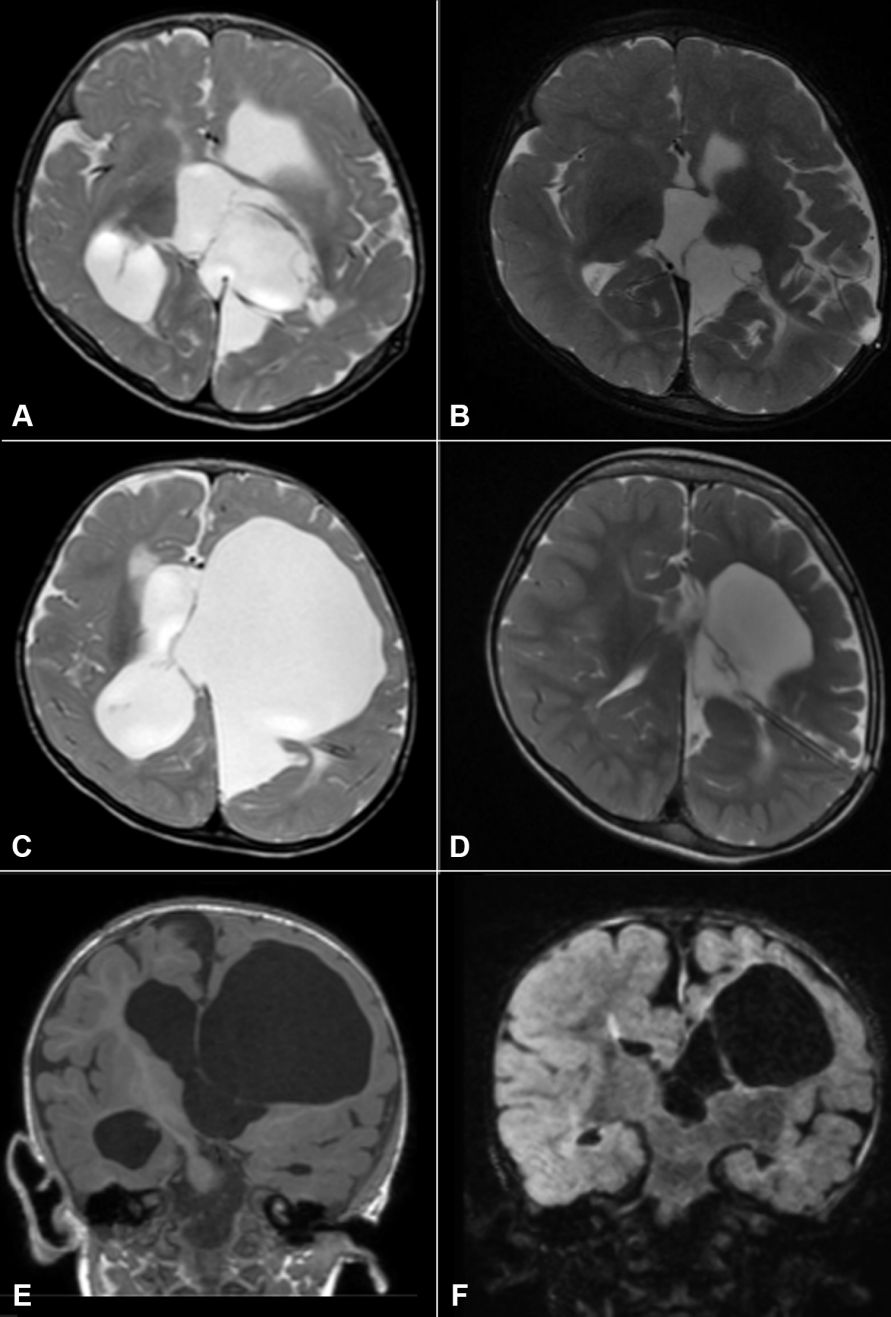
Axial T2-weighted (A–D) and coronal T1-weighted (E–F) MRI sequences of a patient with multicystic hydrocephalus. Various septation create several different compartments within the ventricular system and a significant part of brain parenchyma is lost. Form preoperative (A, C, E) to postoperative (B, D, F) images a reduction of the ventricular loculation is visible
